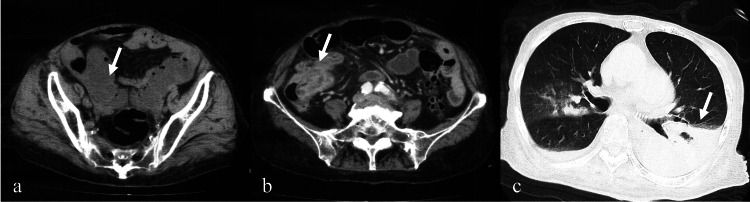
Abdominal and chest computed tomography of case 1. Abdominal computed tomography (CT) revealed small intestine dilation and intestinal effusion, indicating intestinal obstruction (a, arrow). Contrast-enhanced CT displayed a contrast-enhanced mass shadow from the terminal ileum to the proximal colon (b, arrow), with dilation of the intestinal tract. A chest CT performed 19 months after surgery revealed pneumonia and pleural effusion without apparent lung metastasis (c, arrow).
